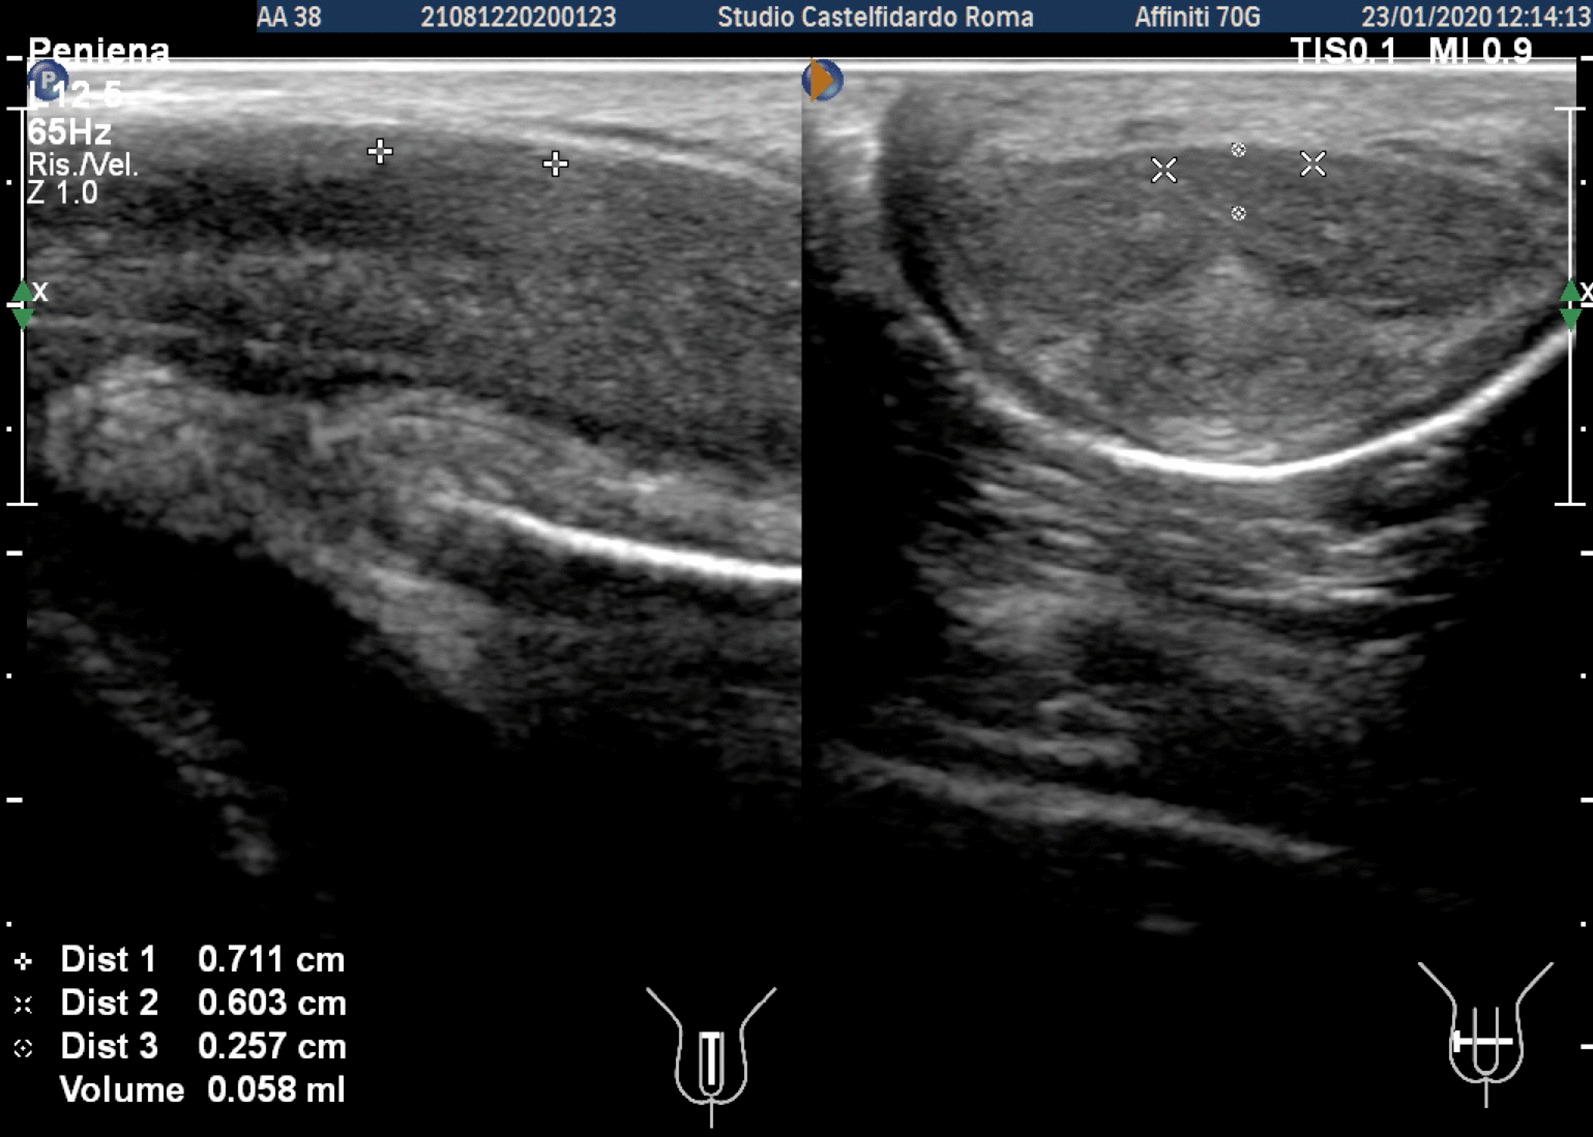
Penile ultrasound after the second treatment cycle (longitudinal and transverse scan)
